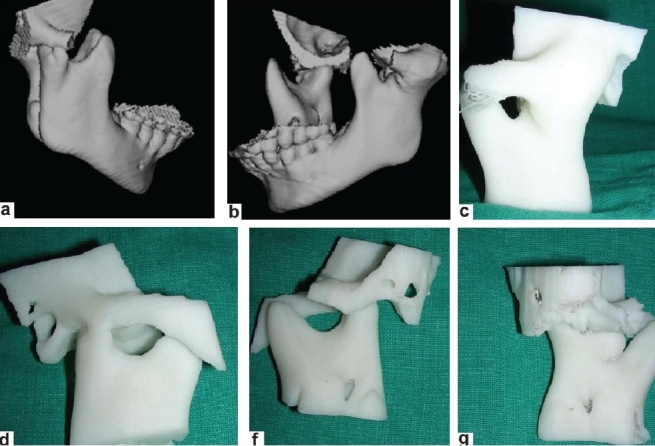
(a-b) Three-dimensional computed tomography scan of the patient; (c-g): From these computed tomography images, an SLE model is made by the rapid prototypic technique
